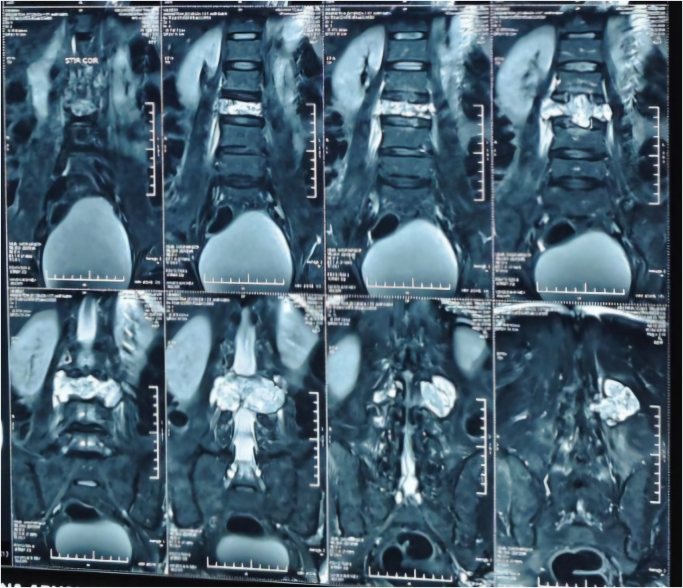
MRI coronal views of the lumbar spine and pelvis showing a heterogeneous bony mass arising near the vertebrae, displacing adjacent soft tissues. The lesion appears lobulated and dense, suggesting a possible osteogenic origin. MRI, magnetic resonance imaging.
